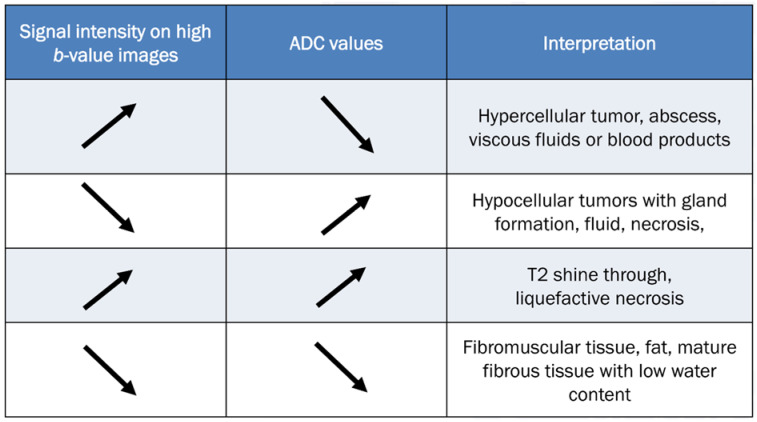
Guidelines for interpreting DWI [2,69]. The table provides interpretation based on the increase in *b*-values and the corresponding ADC map. The direction of the arrow indicates increase and decrease of signal intensity.
